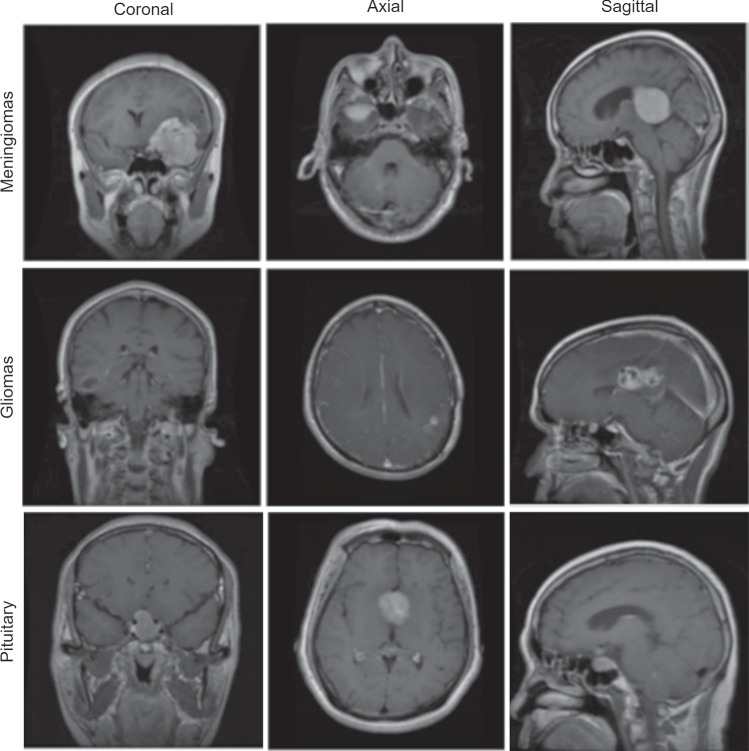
In the Figshare dataset, MRI images of meningiomas, gliomas, and pituitary tumors are presented through sagittal, coronal, and axial cross-sections [14]
